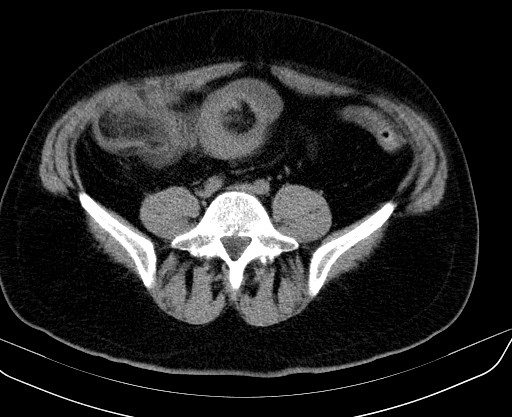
An abdominal computed tomography scan shows a 'sausage'-shape soft-tissue mass in the ascendant colon and thickened walls of the ileum.
